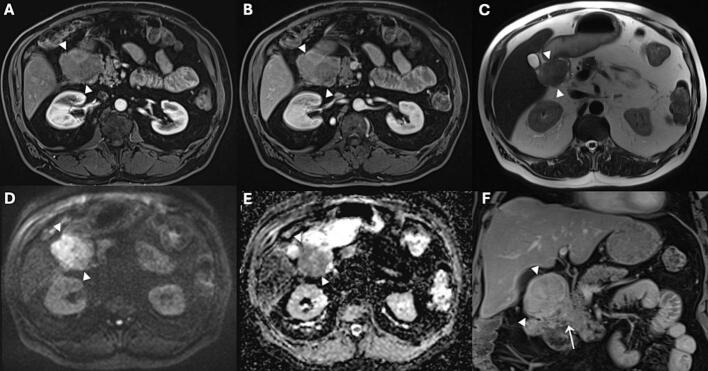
Abdominal MRI in axial (A-E) and coronal (F) direction. A mass in the proximal duodenum (arrowheads) is visible, with only slight heterogeneous enhancement in the late arterial (A) and portal venous phase (B and F), heterogeneous slightly hyperintense signal intensity on T2 weighted imaging (C), and clear diffusion restriction (D and E). The lesion extends into the fatty tissue surrounding the duodenum as well as in the pancreaticoduodenal groove (arrow in F).
